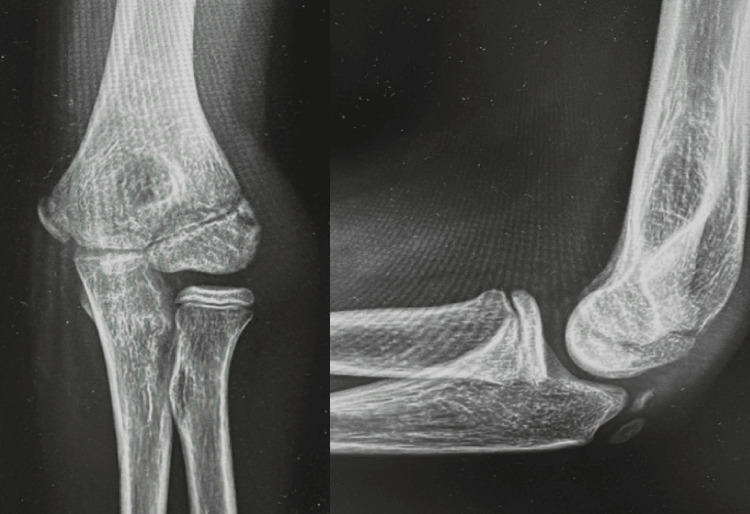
Left elbow radiographs: one-year postoperative follow-up Anteroposterior and lateral views of the elbow.
